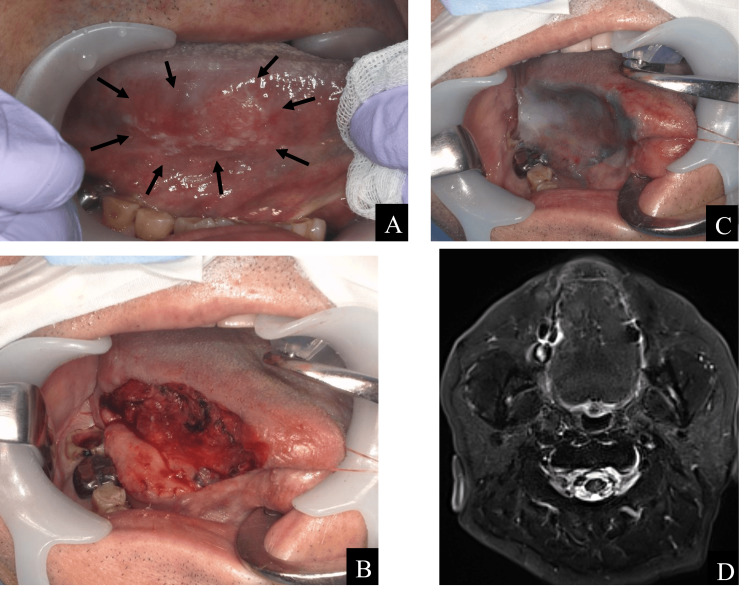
The treatment process for tongue cancer. (A) Intraoral photograph at the initial visit. The diagnosis was tongue cancer T2N0M0, with a long diameter of 21 mm and a depth of invasion of 2.6 mm. (B) Intraoperative photograph of partial resection of tongue cancer. Intraoperative rapid diagnosis of negative margins was obtained. (C) Polyglycolic acid (PGA) sheets and fibrin glue were used to cover wounds after partial tongue cancer resection. (D) Contrast-enhanced MRI, T2-weighted image, three months after tongue cancer surgery. There were no obvious recurrence findings.
